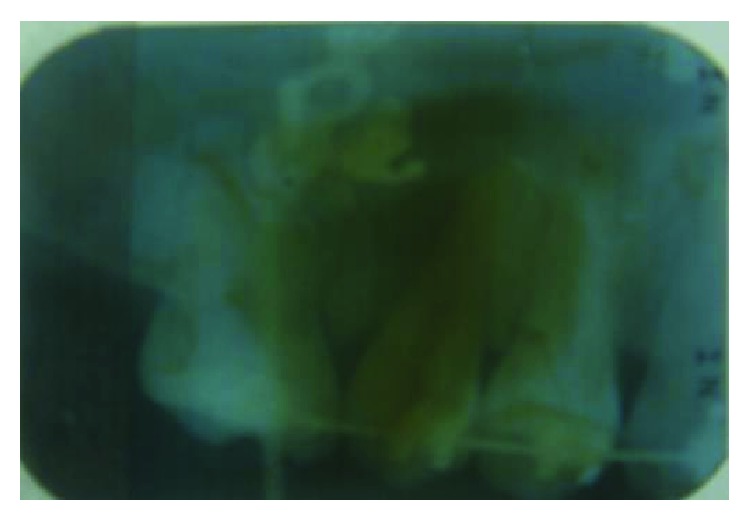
Periapical radiograph with gutta-percha (GP) in situ which had been inserted through the sinus. The GP pointing towards the area of plate and screws with the dislodged molar tube in its vicinity.
